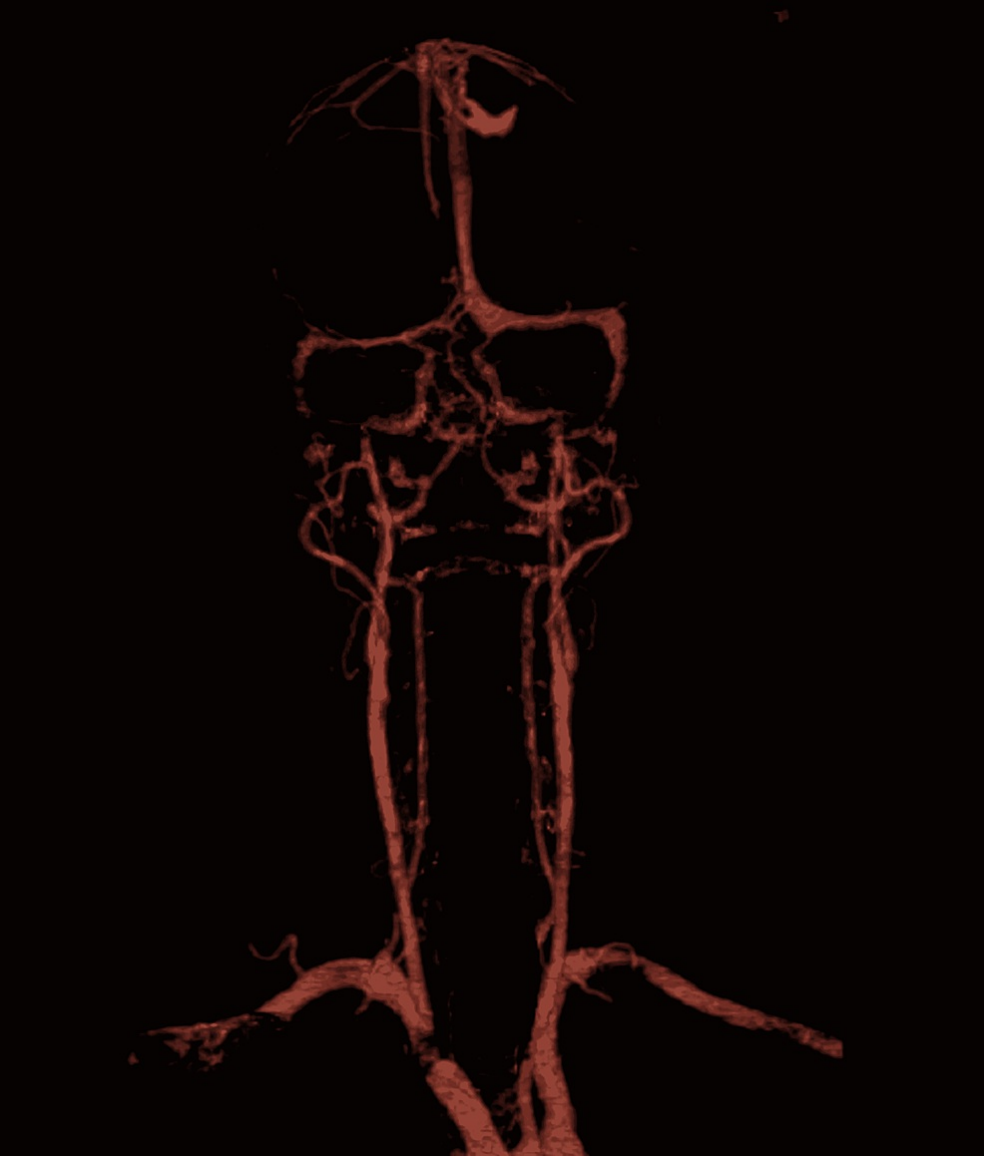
CTA of the next showing no large vessel (intracranial or extracranial) occlusion. No hemodynamically significant arterial stenosis CTA: computed tomography angiography
